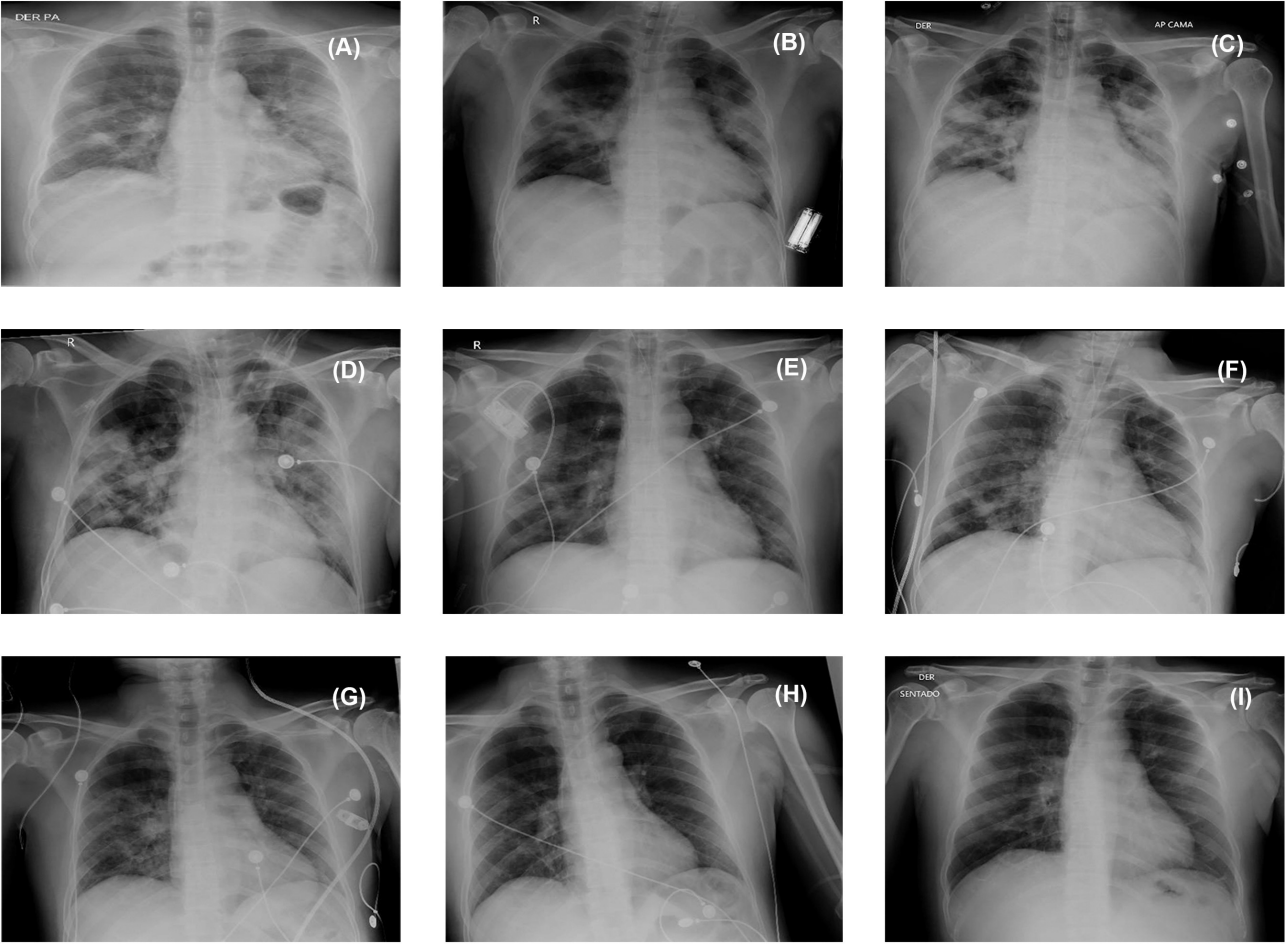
Chest radiographs. The radiographs show bilateral ground-glass opacities (A) on admission to the hospital on March 3, 2020; (B) during intubation on March 5; (C) during begin treatment with steroids on March 6; (D) on entering the intensive care unit on March 9; (E) on March 12; (F) on March 17; and (G) on March 20. No alveolar infiltrates observed on the radiographs (H) on March 23 and (I) during discharge from intensive care unit on March 24, 2020.
